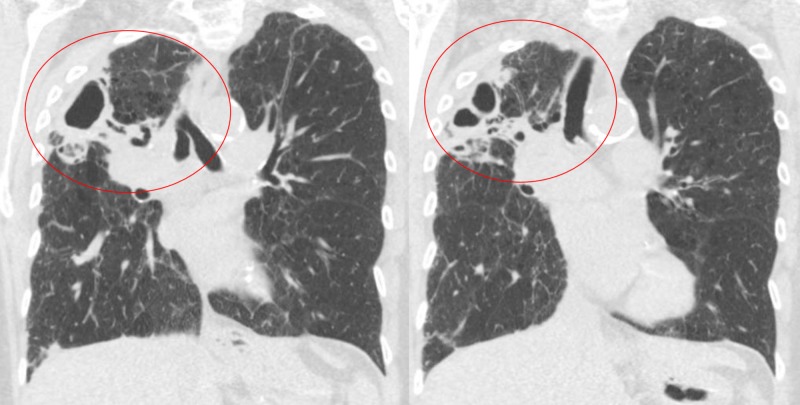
Initial coronal computed tomography of the chest showing right upper lobe cavitary lesion (circled)
